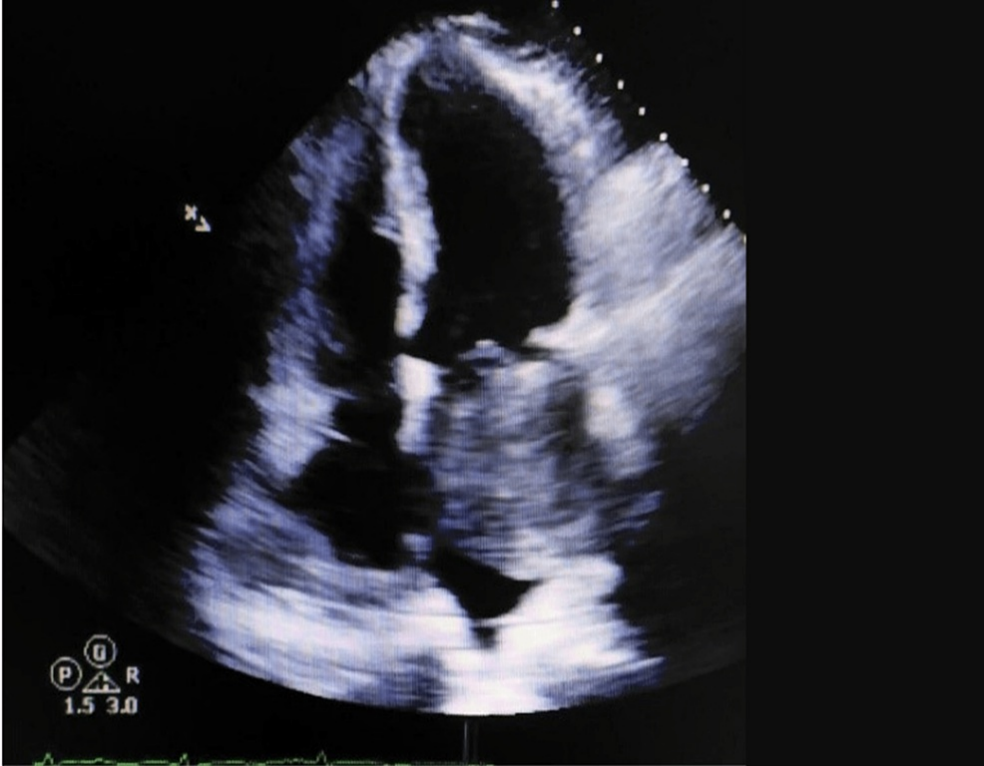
Transthoracic echo with left atrial mass
